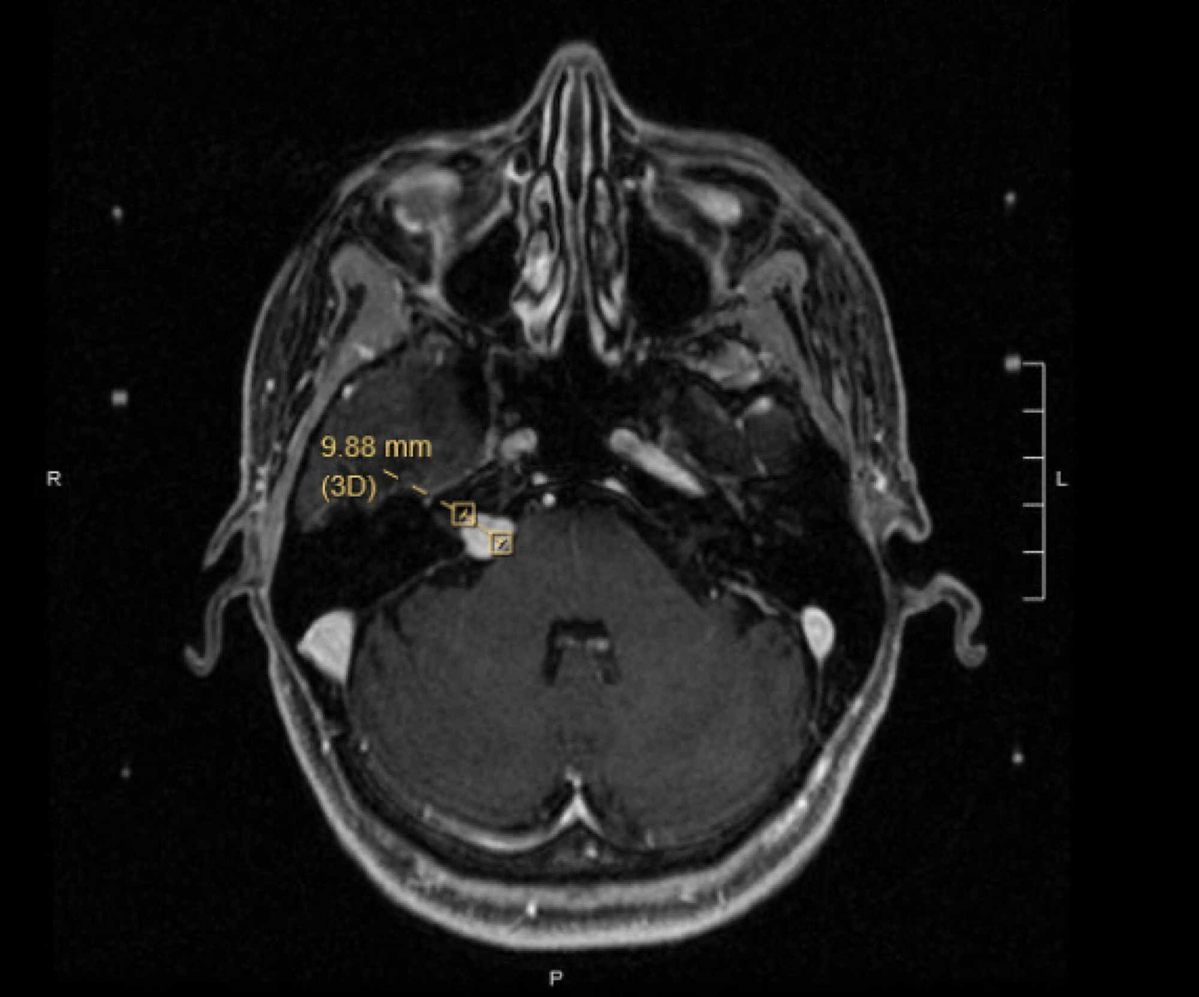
Pre-stereotactic radiosurgery MRI Axial T1 post-contrast image shows a right-side cerebellopontine angle tumor measuring 11 x 12 x 9 mm.
